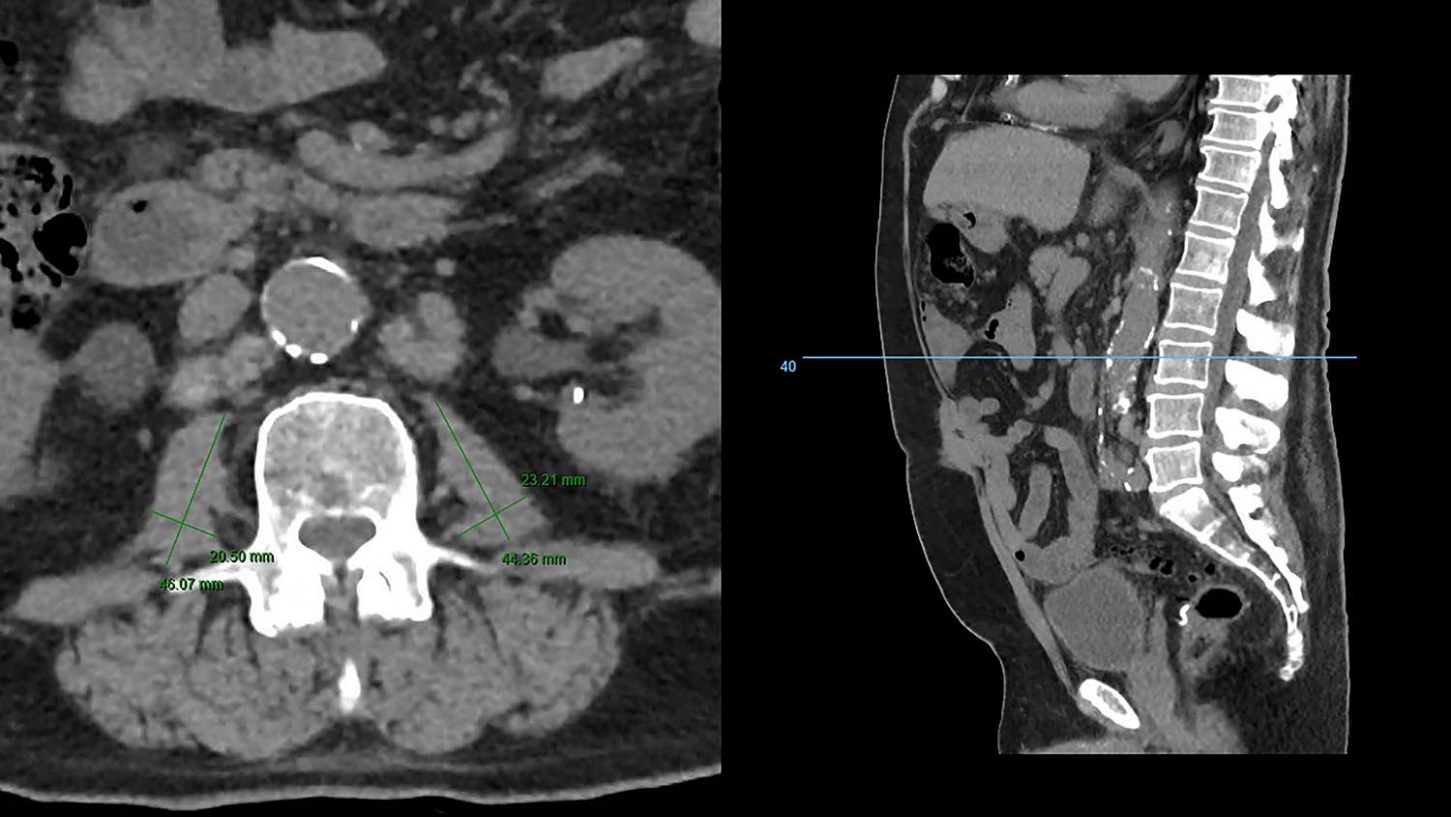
CT scans demonstrating total psoas area calculation at L3 vertebral level
